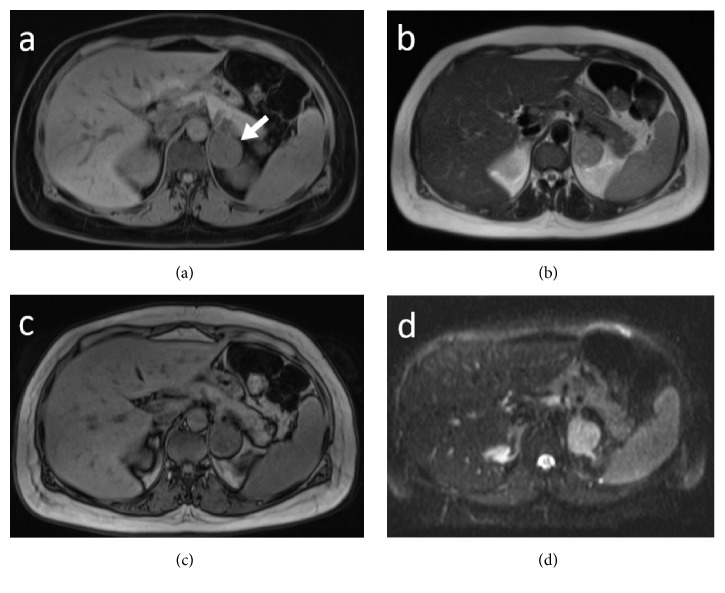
Axial T1-weighted (a) with fat suppression and T2-weighted (b) MR images show a left adrenal mass (arrow in (a)). The mass has heterogeneous high signal intensity on the T2-weighted image, low signal intensity on T1-weighted image, and no signal dropout on opposed phase T1-weighted image (c). Axial diffusion-weighted image (DWI) shows high signal intensity due to restricted diffusion (d).
